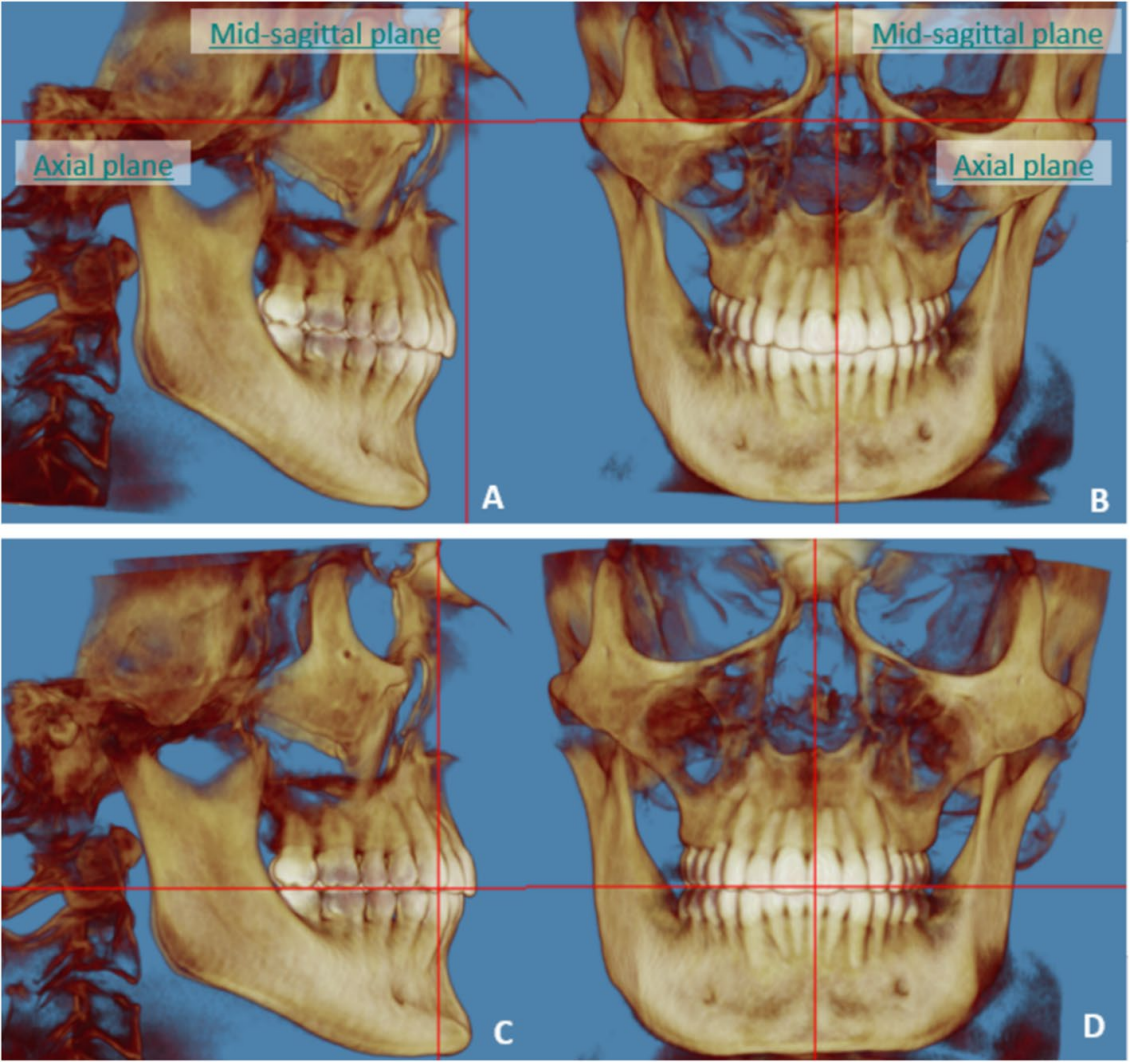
CBCT images were oriented with the midsagittal plane aligned to nasion perpendicular (A-D) and the axial plane aligned to either FHP (A-B) or occlusal plane (C-D) to consistently measure the selected parameters that are related to each orientation
